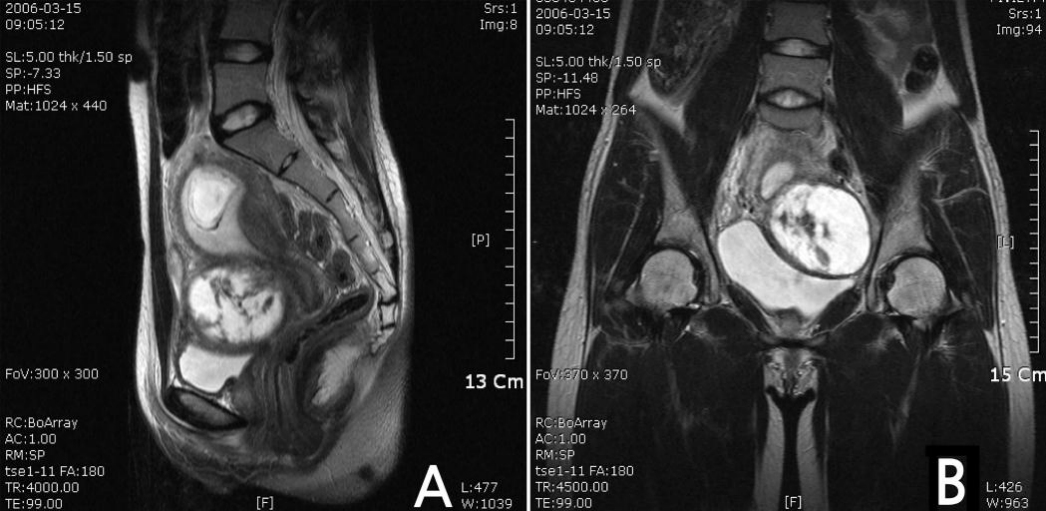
T2-weighted image of MRI findings showed an 8×7×6 cm cystic and solid mass with septa. (A) Sagital view, (B) Coronal view.
